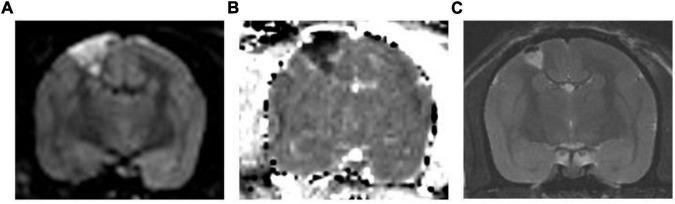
Representative MR Images. (A) Diffusion-weighted image and (B) corresponding apparent diffusion coefficient map. The high-intensity area in the diffusion-weighted image shows low intensity in the apparent diffusion coefficient map, which suggests that this area is infarcted. (C) T2-weighted image 1 month after surgery. The infarcted area shows hyperintensity in the T2-weighted image.
